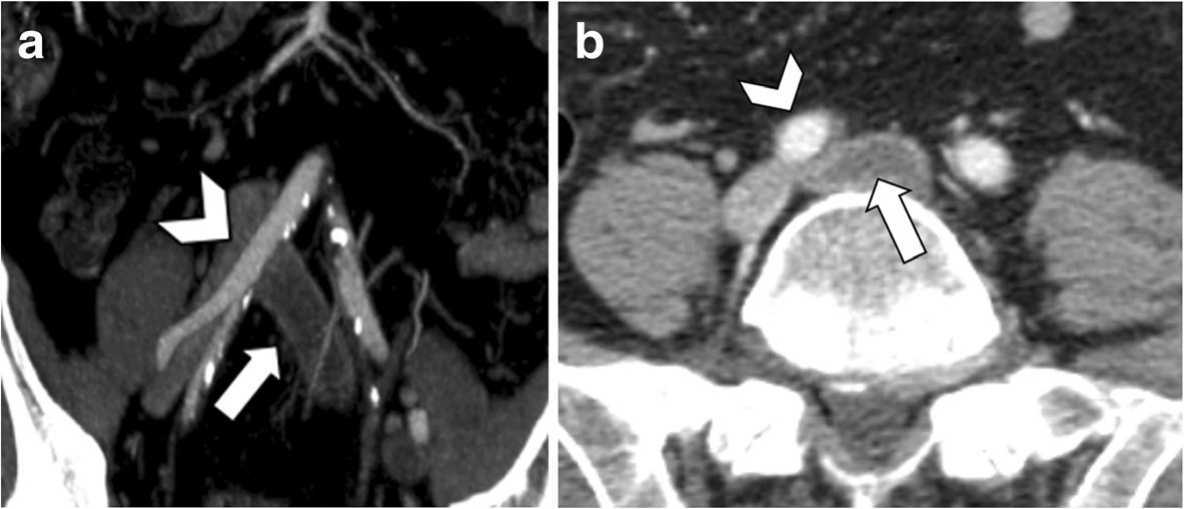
An 85-year-old man with May-Thurner syndrome. Coronal (a) and axial (b) contrast-enhanced CT images show thrombosed left common iliac vein (arrows) compressed between the right common iliac artery (arrowheads) and fifth lumbar vertebra
